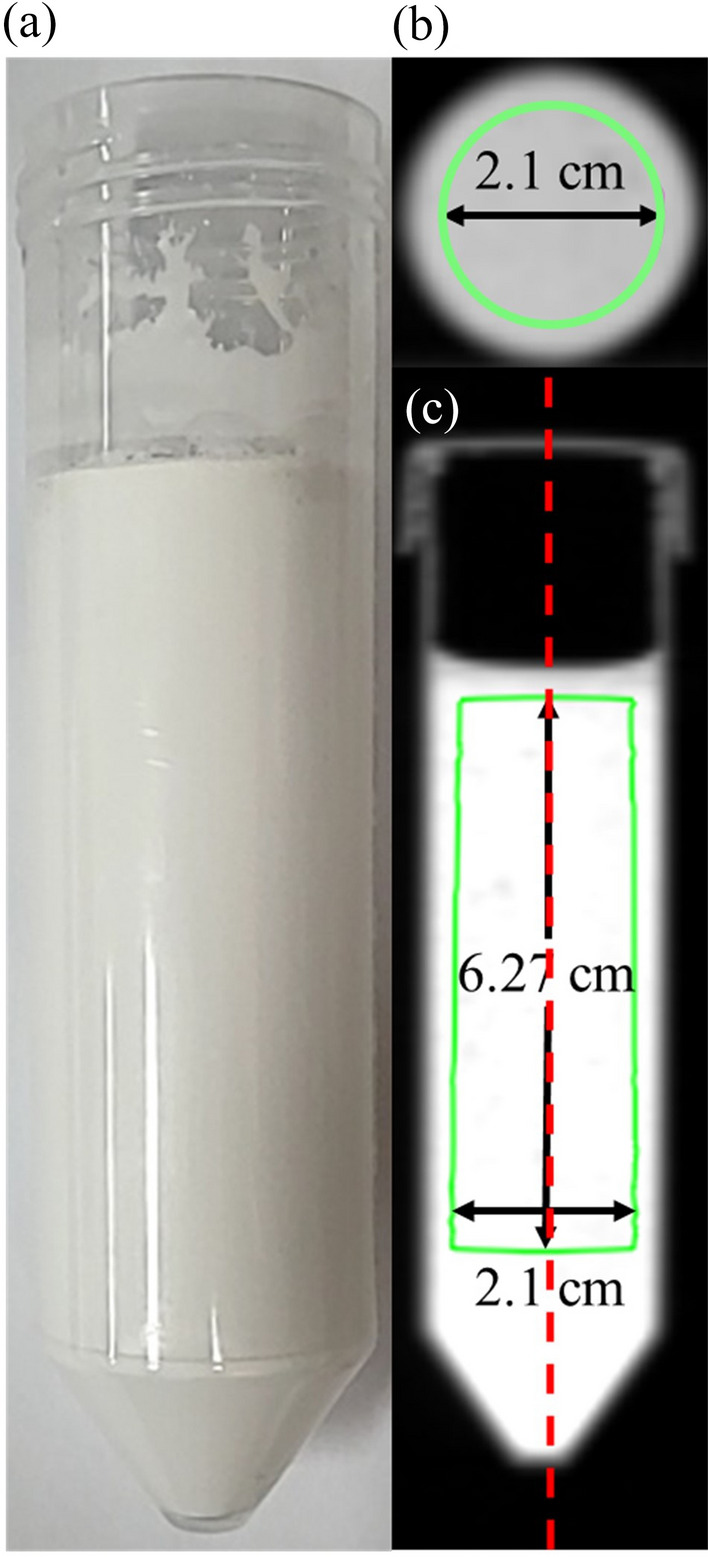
(a) Photograph of the mixture of plaster and PLA power. (b) Transversal and (c) frontal CT images of the samples.
